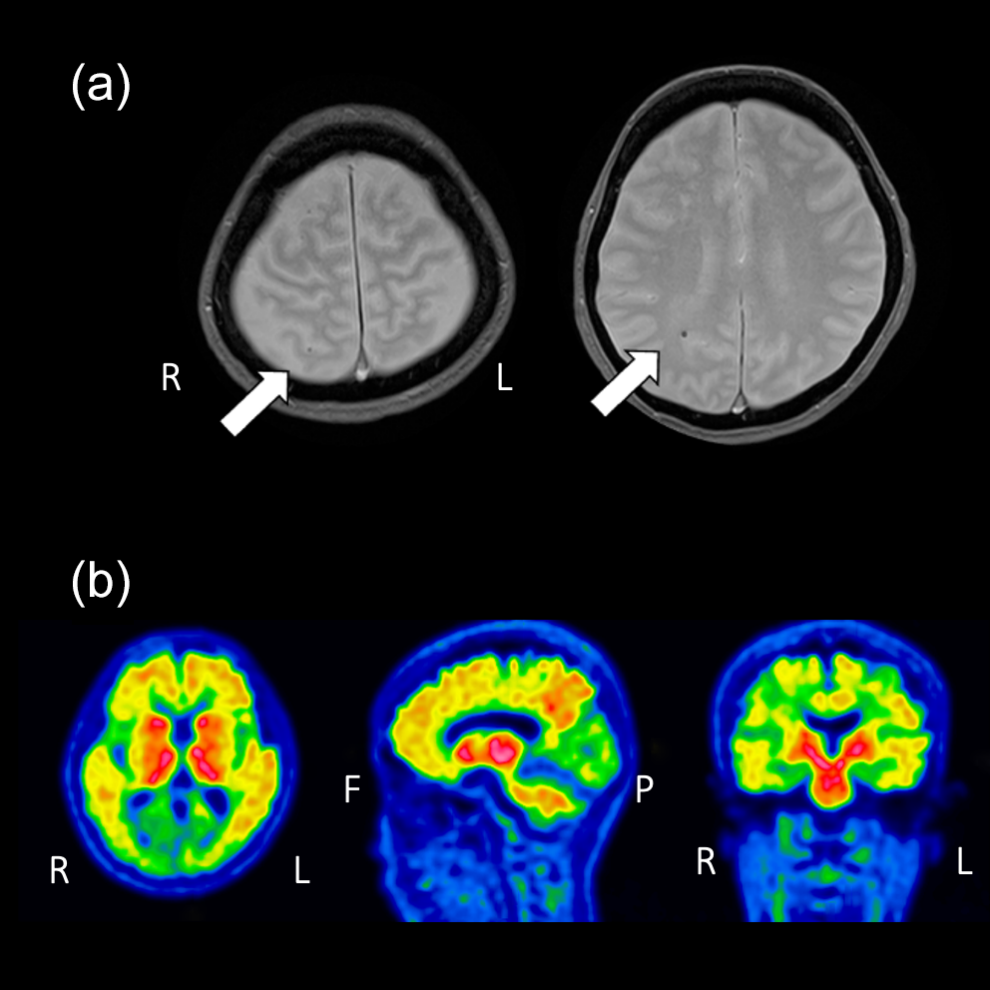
Neuroimaging results pre‐lecanemab/amyloid‐related imaging abnormalities (ARIA). Two microbleeds are identified using T_2_* magnetic resonance imaging (MRI) (a). Positron emission tomography imaging using ^18^F‐flutemetamol showed amyloid β plaque deposition in the frontal, lateral temporal and parietal lobe, posterior cingulate gyri, praecuneus, and striatum (b). The white arrows show microbleeds.
